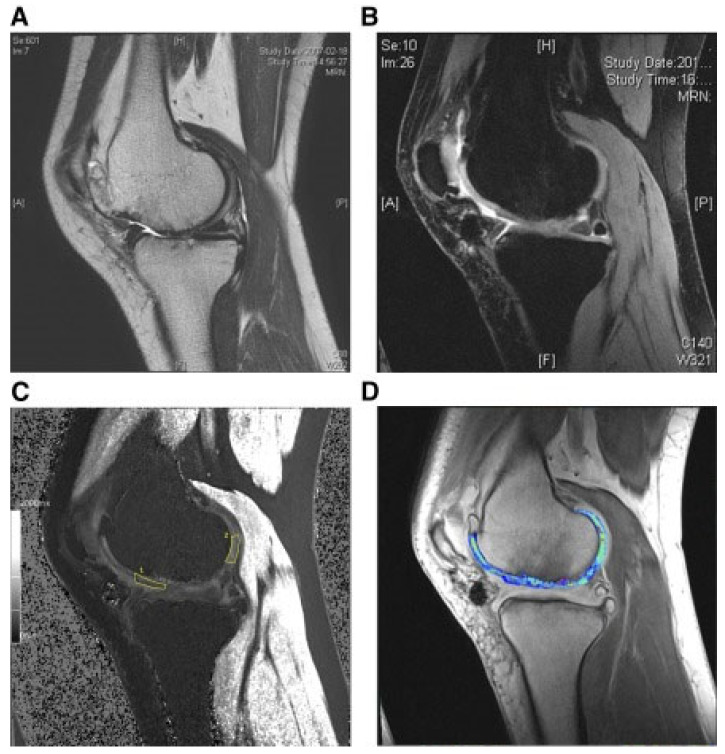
MRI evaluation of cartilage regeneration 3 years after HUCB-MSC treatment. (A) A preoperative cartilage defect. (B) Cartilage regeneration at 3 years post-transplantation. (C) The change in relative cartilage relaxation rate was calculated by sampling in the marked area. (D) An increased GAG content is shown in relation to the blue signal. Reprinted from [118]. MRI, magnetic resonance imaging; HUCB-MSCs, human umbilical cord blood-derived mesenchymal stem cells; GAG: glycosaminoglycan.
